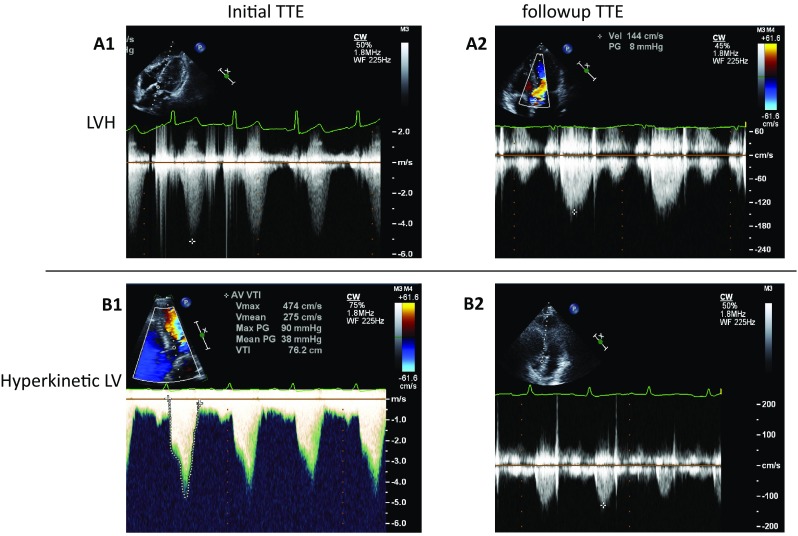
Examples of left ventricular outflow on continuous-wave Doppler echocardiography at initial and follow-up TTE in cases with LVH and hyperkinetic LV compared with LVOT PG (A1: 106 mmHg in a case with LVH, B1: 90 mmHg in a case with hyperkinetic LV) at the initial TTE, LVOT PG (A2: 8 mmHg in a case with LVH, B2: 7 mmHg in a case with hyperkinetic LV) at follow-up TTE showed decreases. *TTE* transthoracic echocardiography, *LVH* left ventricular hypertrophy, *LV* left ventricle, *LVOT PG* left ventricular outflow tract pressure gradient
